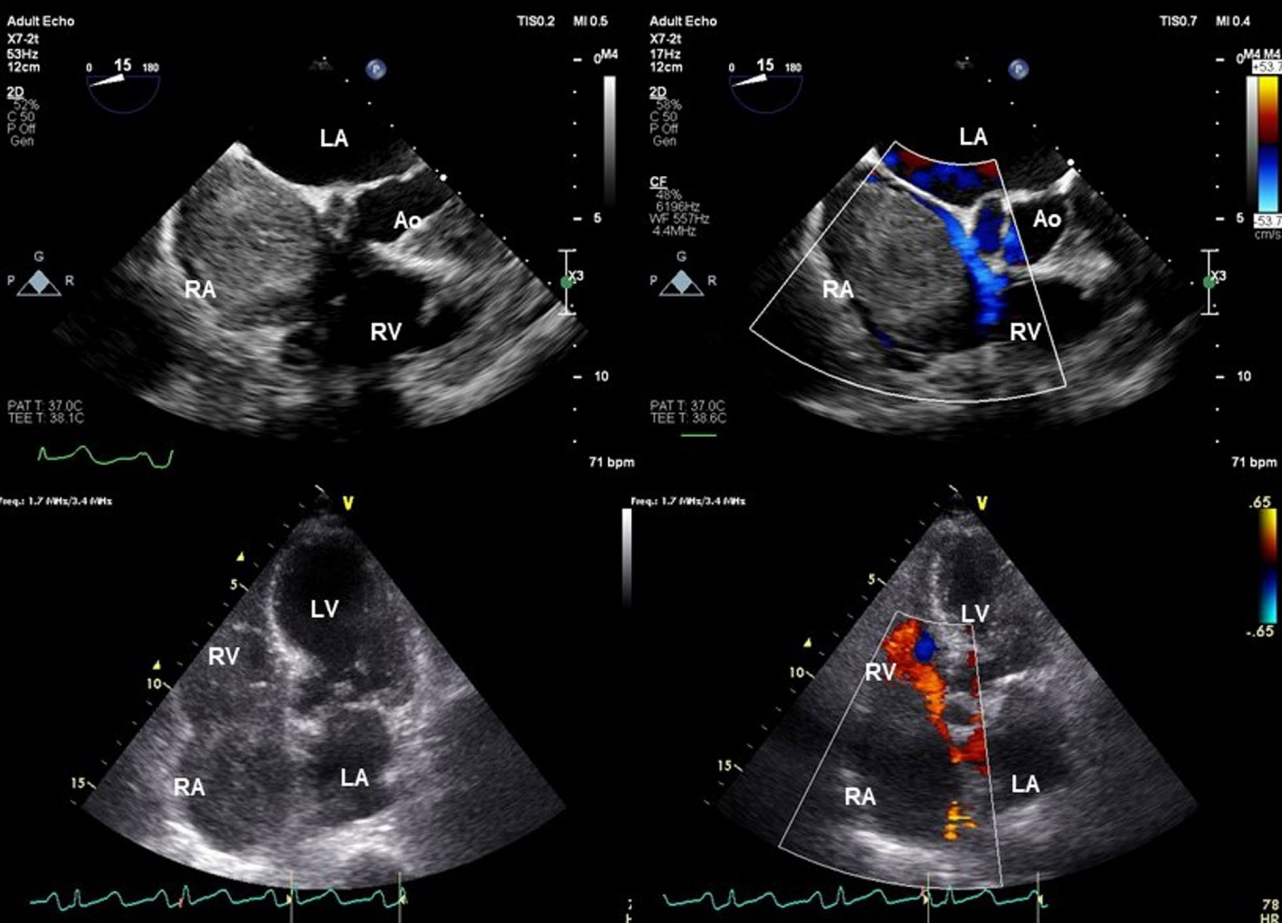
Transthoracic and transesophageal cardiac ultrasonography demonstrate a large mass nearly filling the right atrium. *RA* right atrium, *RV* right ventricle, *LA* left atrium, *LV* left ventricle, *Ao* aorta
